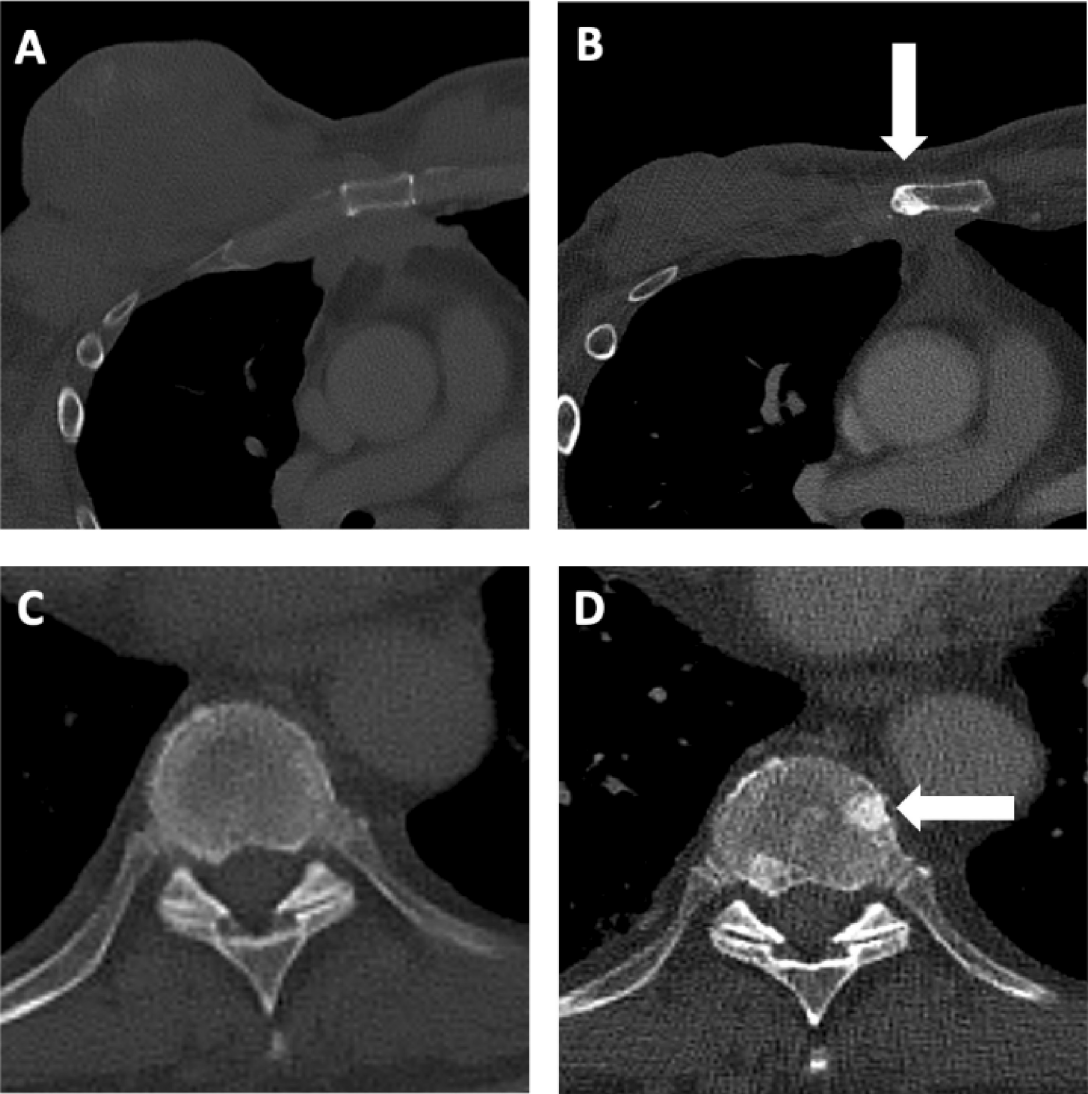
Corresponding to Figure 1, axial bone window images of the identical thoracic CT before (A, C) and 6 months after the initiation of therapy (B, D) are shown. During follow‐up newly defined focal sclerotic bone lesions (arrows) were identified in the corpus sterni on the right adjacent to the sternum and in the ninth thoracic vertebra on the left. In view of the clear size regression of the soft tissue tumor and the lack of evidence of progressive bone metastases, these lesions were classified as an osteoblastic reaction in the course of therapeutic response of sternal infiltration by the soft tissue tumor and previously occult vertebral body metastasis.
